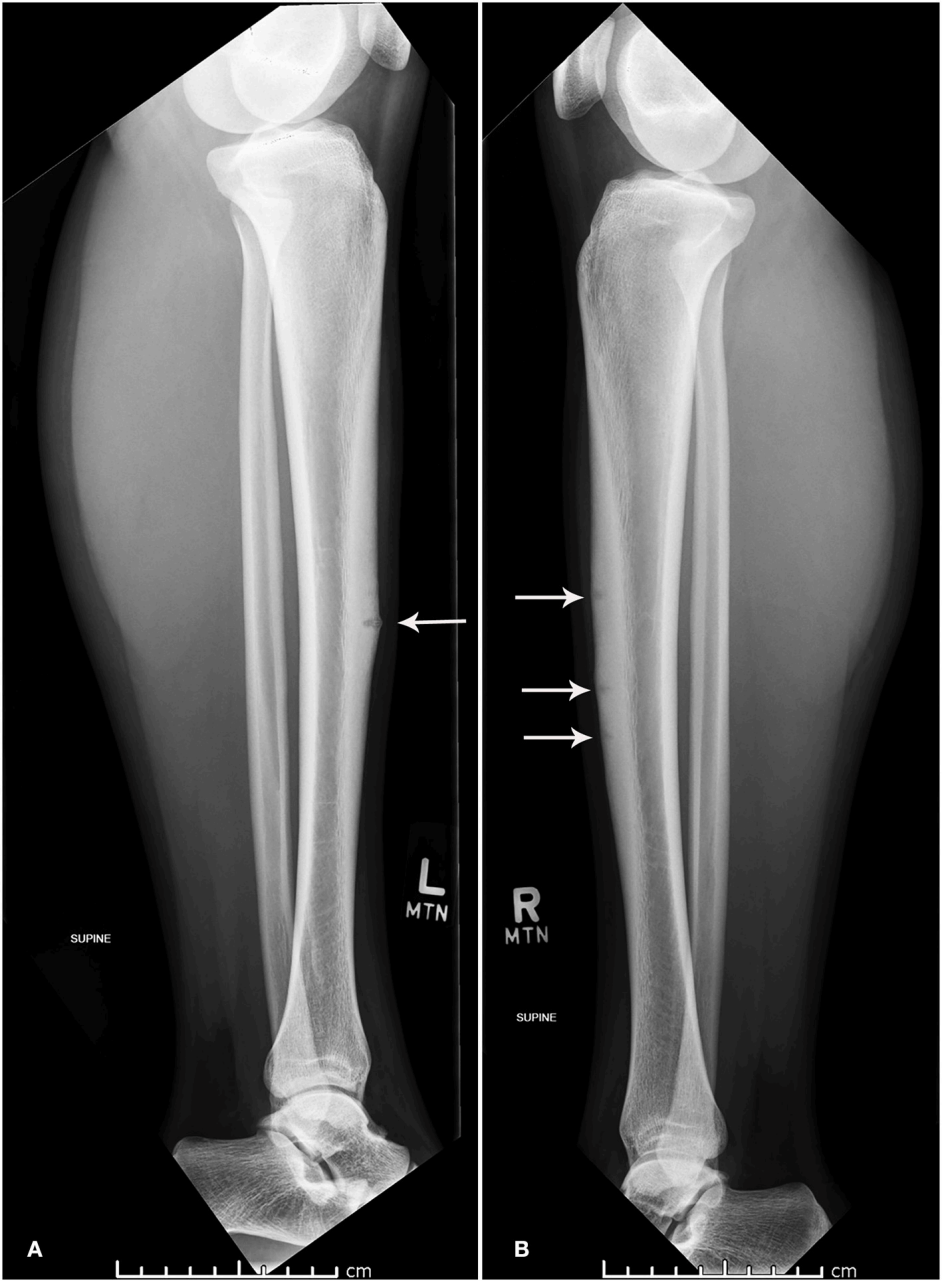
Lateral x-ray of left (A) and right (B) tibia and fibula.
